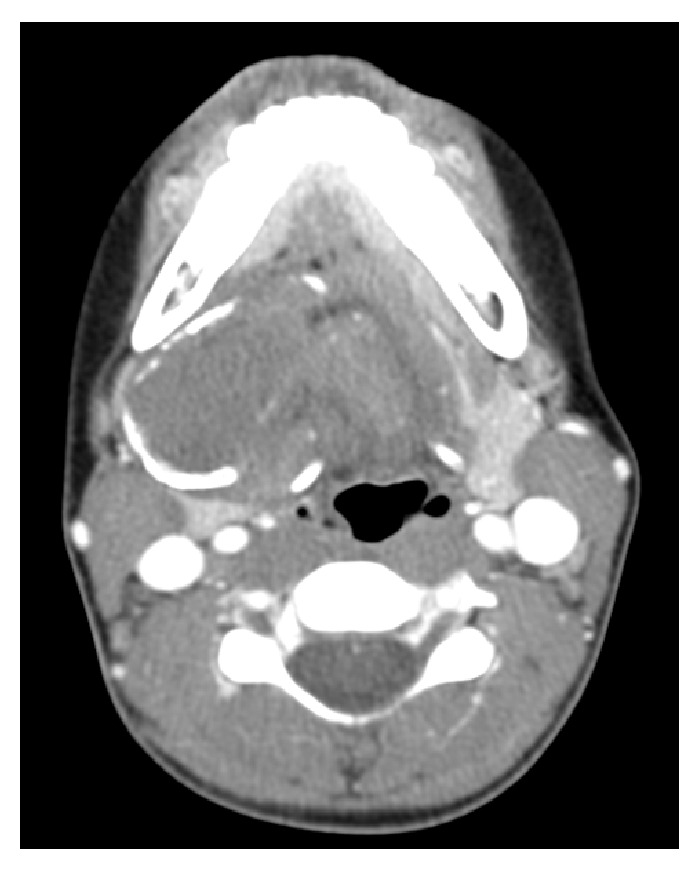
Contrast CT scan showing presence of a heterogeneous, well-defined lesion with peripheral calcification without bone involvement.
